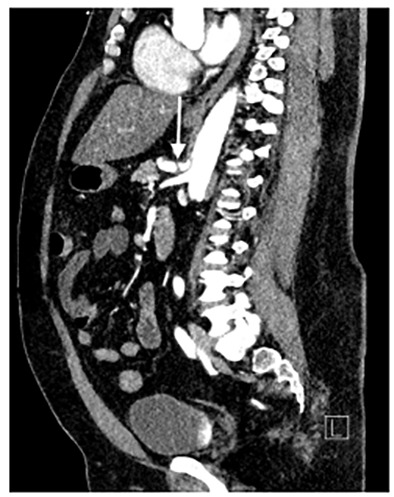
A sagittal image of a contrast-enhanced computed tomography angiogram showing a dissection flap in the celiac trunk (arrow).
